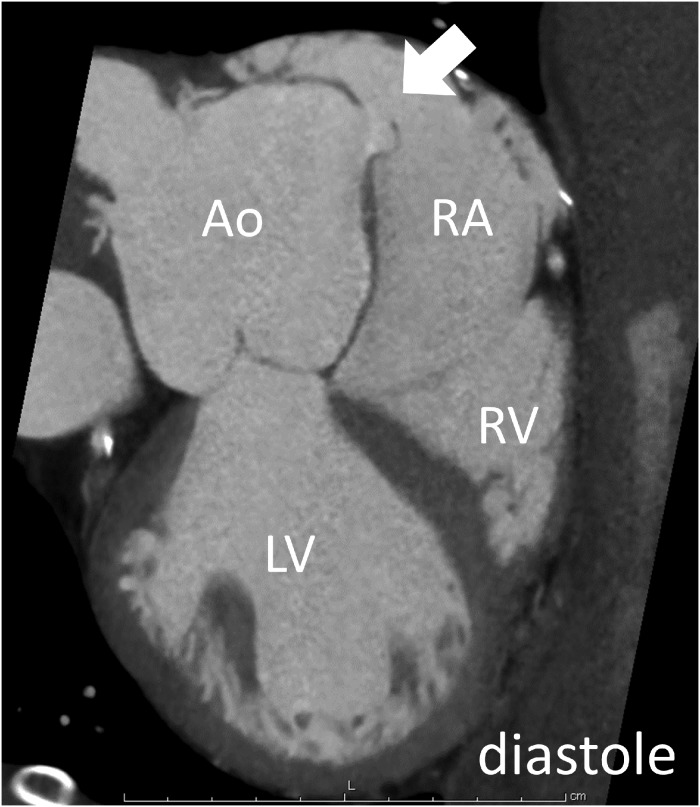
The cine mode reconstruction of cardiac computed tomography showed the flap-type rupture, which was opened to the right atrium in diastole. Ao, aorta; LV, left ventricle; RA, right atrium; RV, right ventricle.
